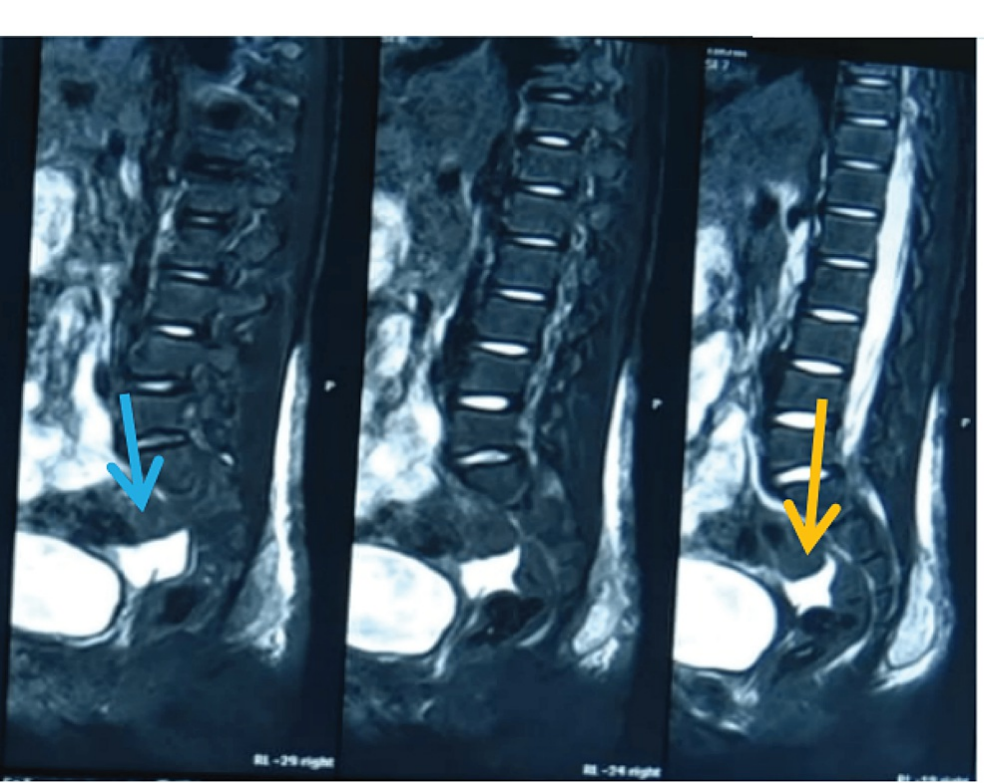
Initial MRI (done one month prior to presentation to our center) revealing evidence of free fluid and collection anterior to sacrum
